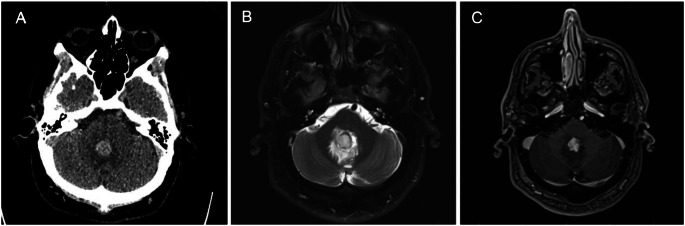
Axial noncontrast CT (A), T2-weighted MRI (B), and postcontrast T1-weighted MRI (C) images through the posterior fossa obtained from a 50-year-old male presenting with clinically suspected hypertensive hemorrhage. The CT image (A) demonstrates a round, hyperattenuating lesion in the cerebellar vermis with surrounding edema. On the subsequent MRI, the lesion demonstrates internal T2 signal hyperintensity (B) and solid internal enhancement (C)
